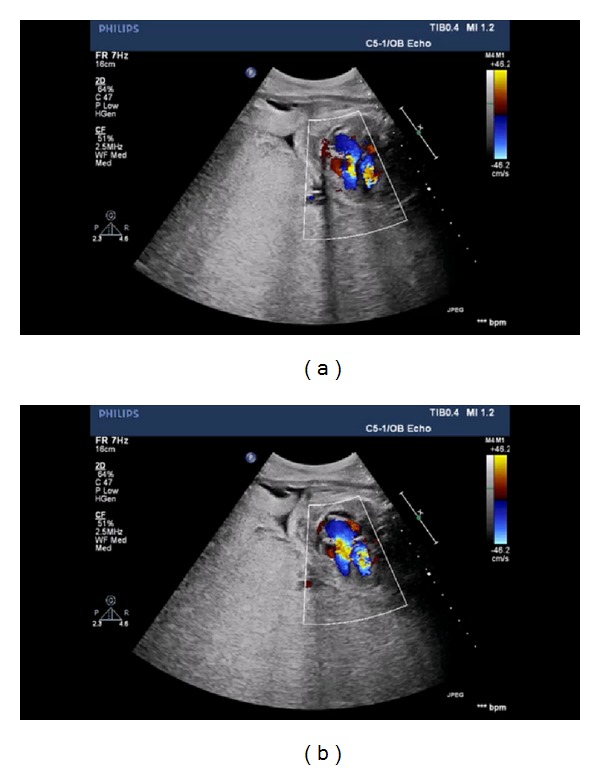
Pulsed wave Doppler examination demonstrating significant tricuspid regurgitation.
